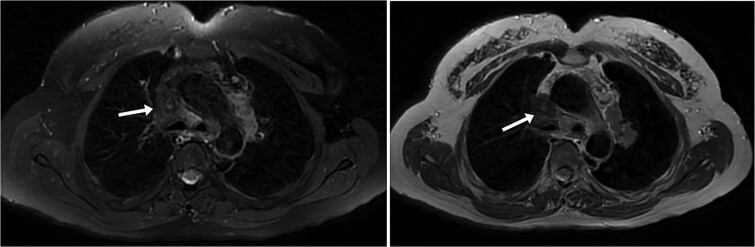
MRI T2-weighted and T2 FS sequences allowing for a more detailed analysis of the parietal thickening of the SVC. It is noted that there is no endoluminal thrombus or compressive lymphadenopathy adjacent to the SVC.
